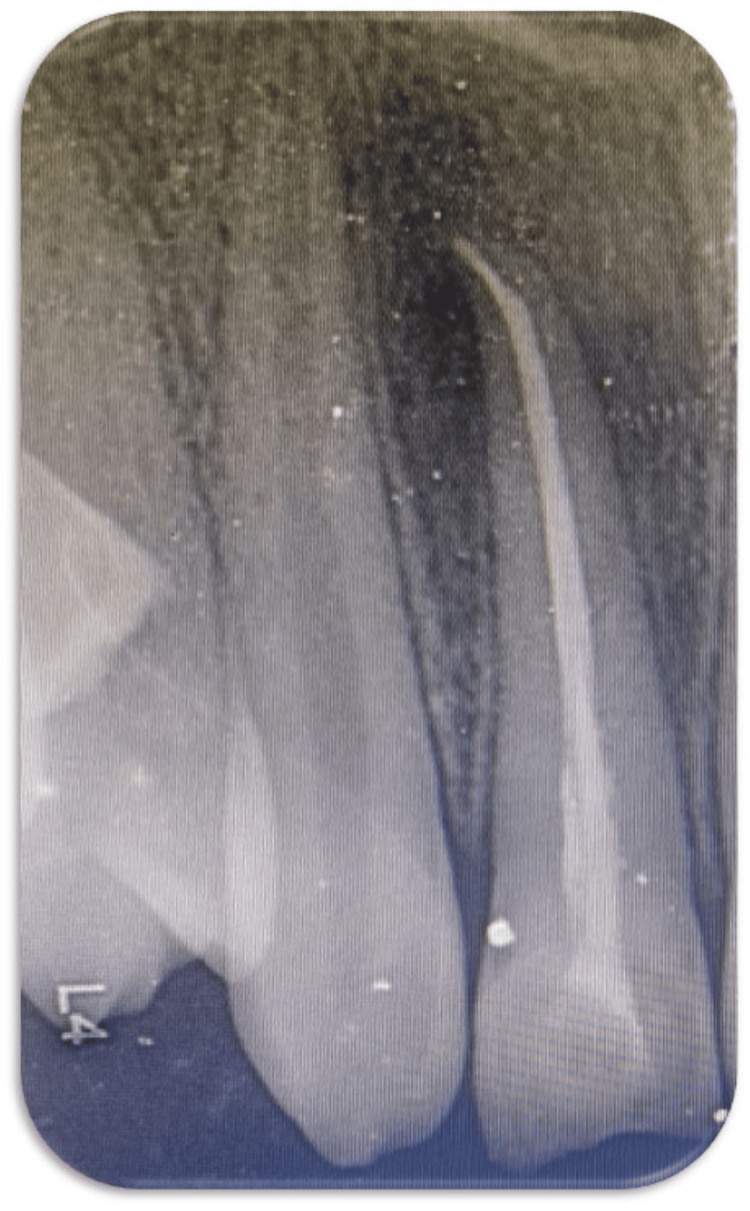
Postoperative radiograph showing root canal treatment with the right permanent maxillary lateral incisor
